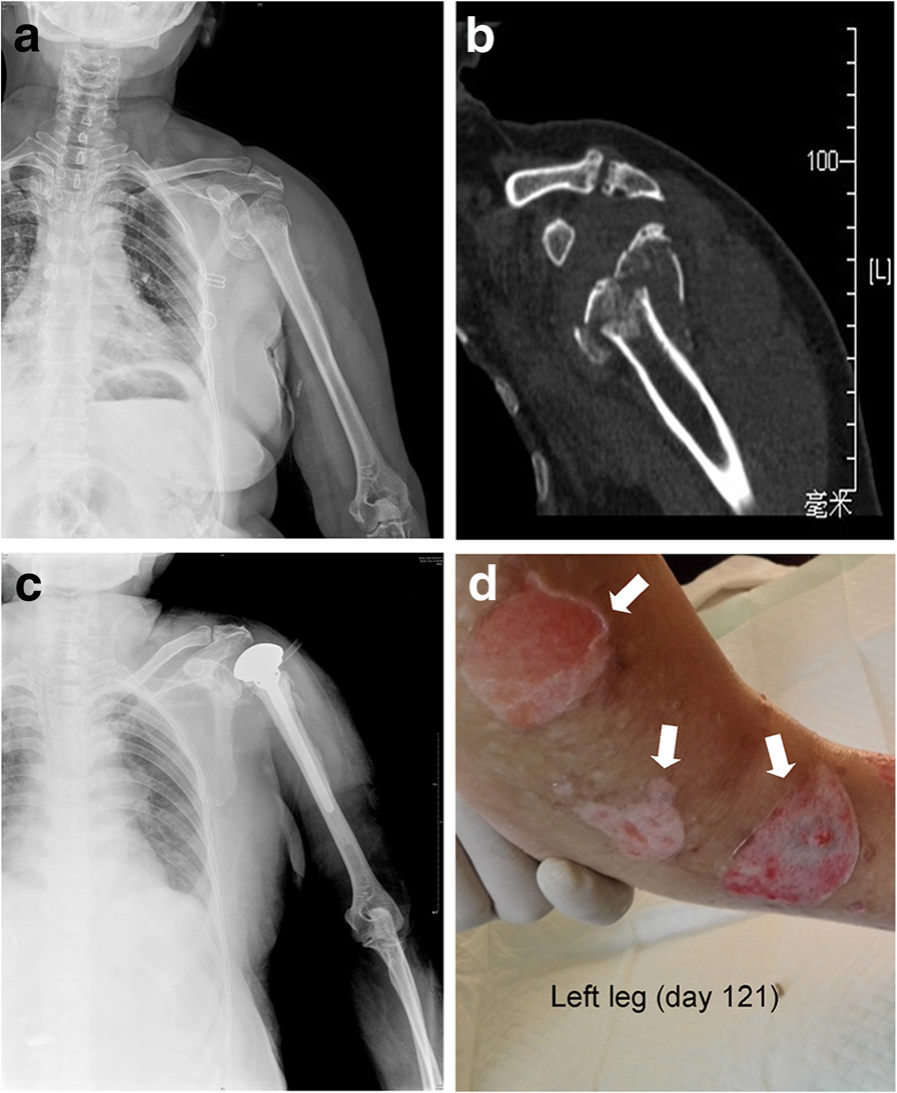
Radiograpic findings of the left shoulder and ruptrured blisters in the left leg. a: Plain radiograph showing the patient’s left shoulder at admission. b: CT scans of the same area. c: Plain radiograph of the left shoulder after hemiarthroplasty surgery. d: During the treatment failure stage, the patient developed multiple blisters in the left leg. Defects in the skin developed after the blisters ruptured (arrows)
